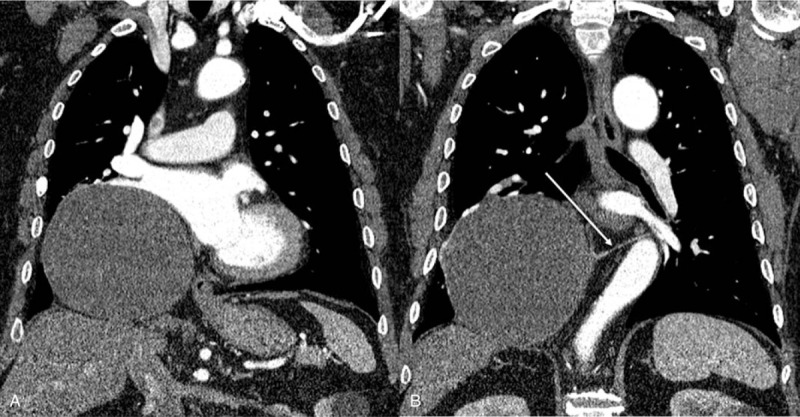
(A and B) Contrast-enhanced chest computed tomography (CT) shows a huge cystic mass measuring 15 cm in the right lower lobe. There is a thin aberrant systemic artery from the descending thoracic aorta (arrow).
